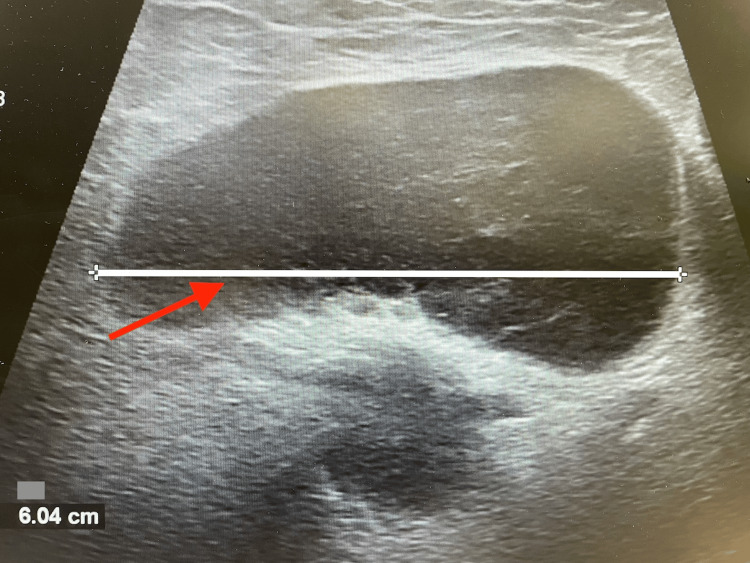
Ultrasound image of an enlarged inguinal lymph node measuring 6.04 cm in diameter
